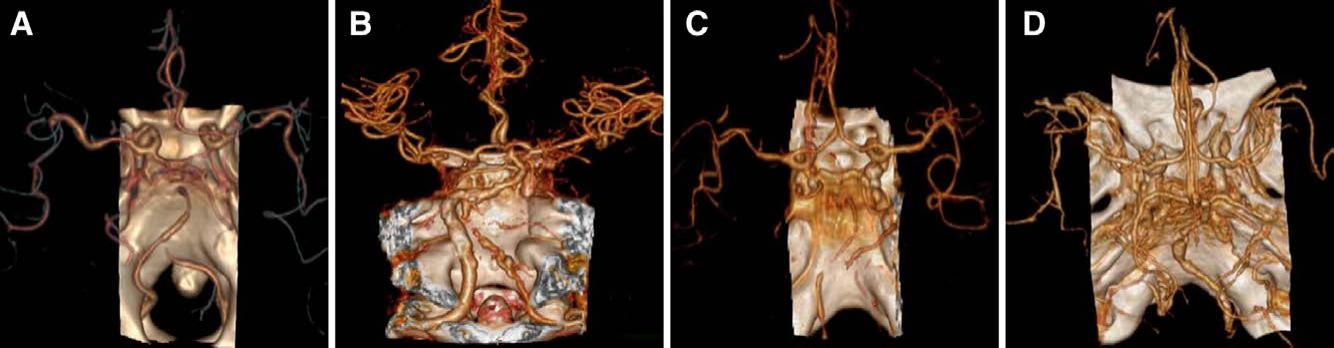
Computed tomography angiography (CTA) scans of the head and neck CTA scans of the head and neck showed severe stenosis or occlusion of the vertebral artery, basilar artery, or posterior cerebral artery.
